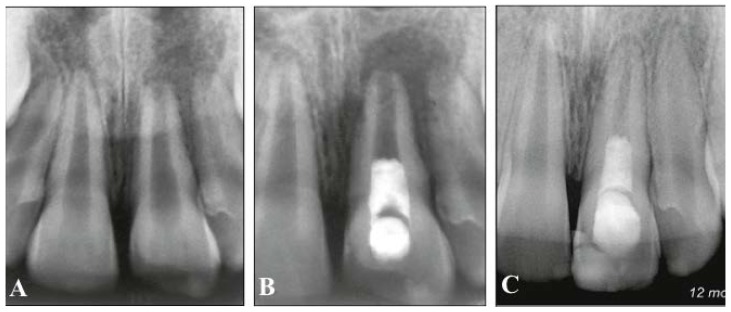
Radiographs of revascularized human immature permanent tooth #9. (A) Preoperative radiograph to show inflammatory periapical lesion; (B) Postoperative radiograph after regenerative endodontic procedures; (C) At 12-month follow-up, thickening of the canal walls and continued root maturation [54].
